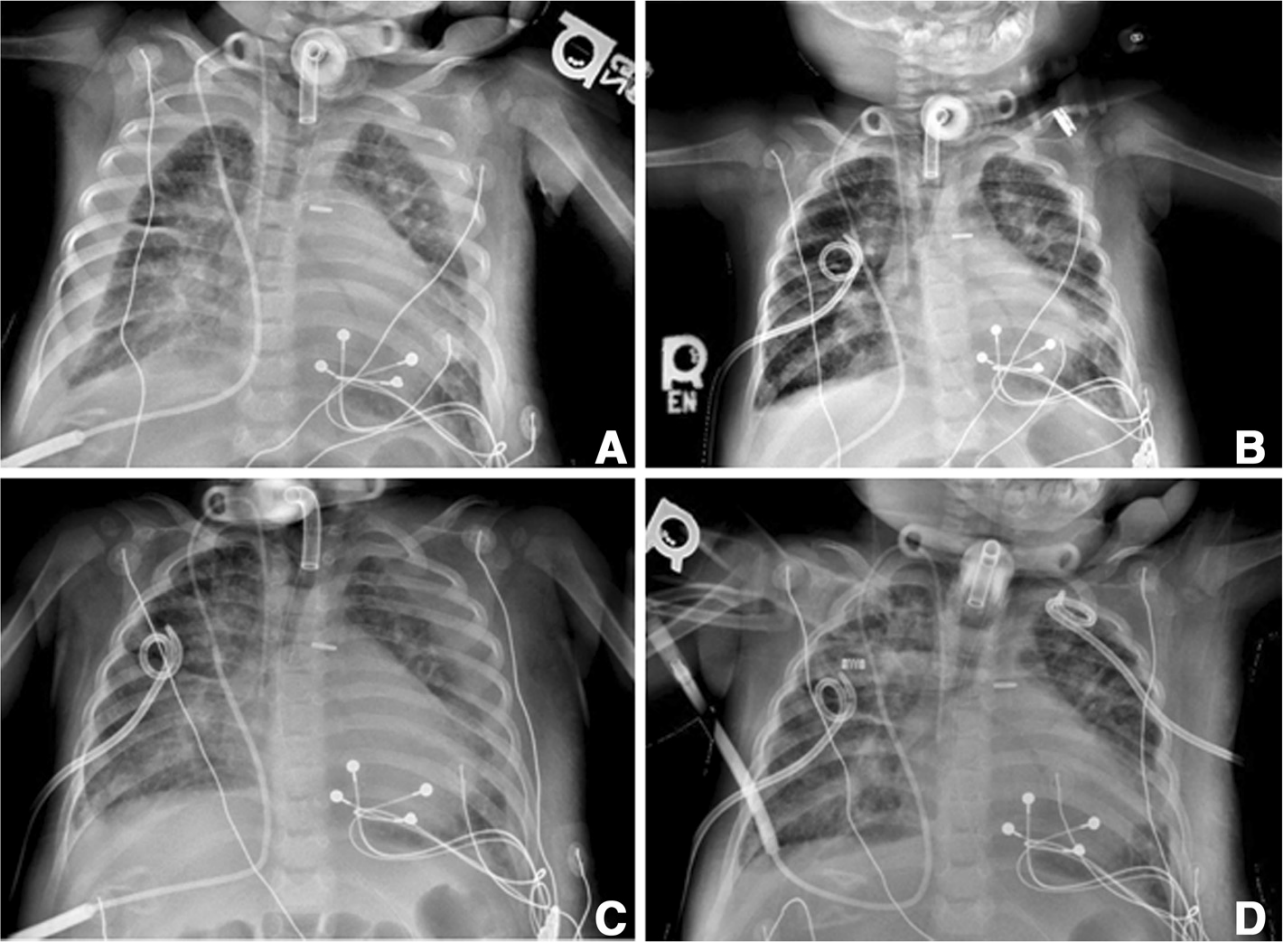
Chest X-ray images of pleural effusions. a Bilateral pleural effusions noted on postoperative day 3. b Following right chest tube placement and left thoracentesis on postoperative day 3. c Reaccumulation of left pleural effusion on postoperative day 4. d Following left chest tube placement on postoperative day 4
